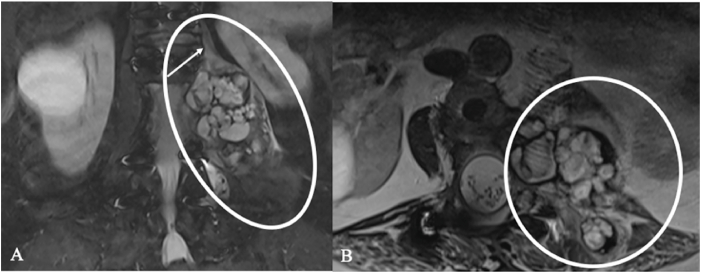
Chordoma in a 72-year-old woman. MRI demonstrates a multilobulated lesion with predominantly high signal on fluid-sensitive sequences and internal septations along the lumbosacral plexus. (A) The coronal image shows the cluster-like mass tracking cranio-caudally (white ellipse; arrow indicates the plexus course). (B) Axial image depicts the conglomerate abutting on the plexus elements (white ellipse). The imaging appearance initially raised suspicion for a peripheral nerve sheath tumor. A partial resection was performed. Sensory and motor deficits were already present preoperatively.
